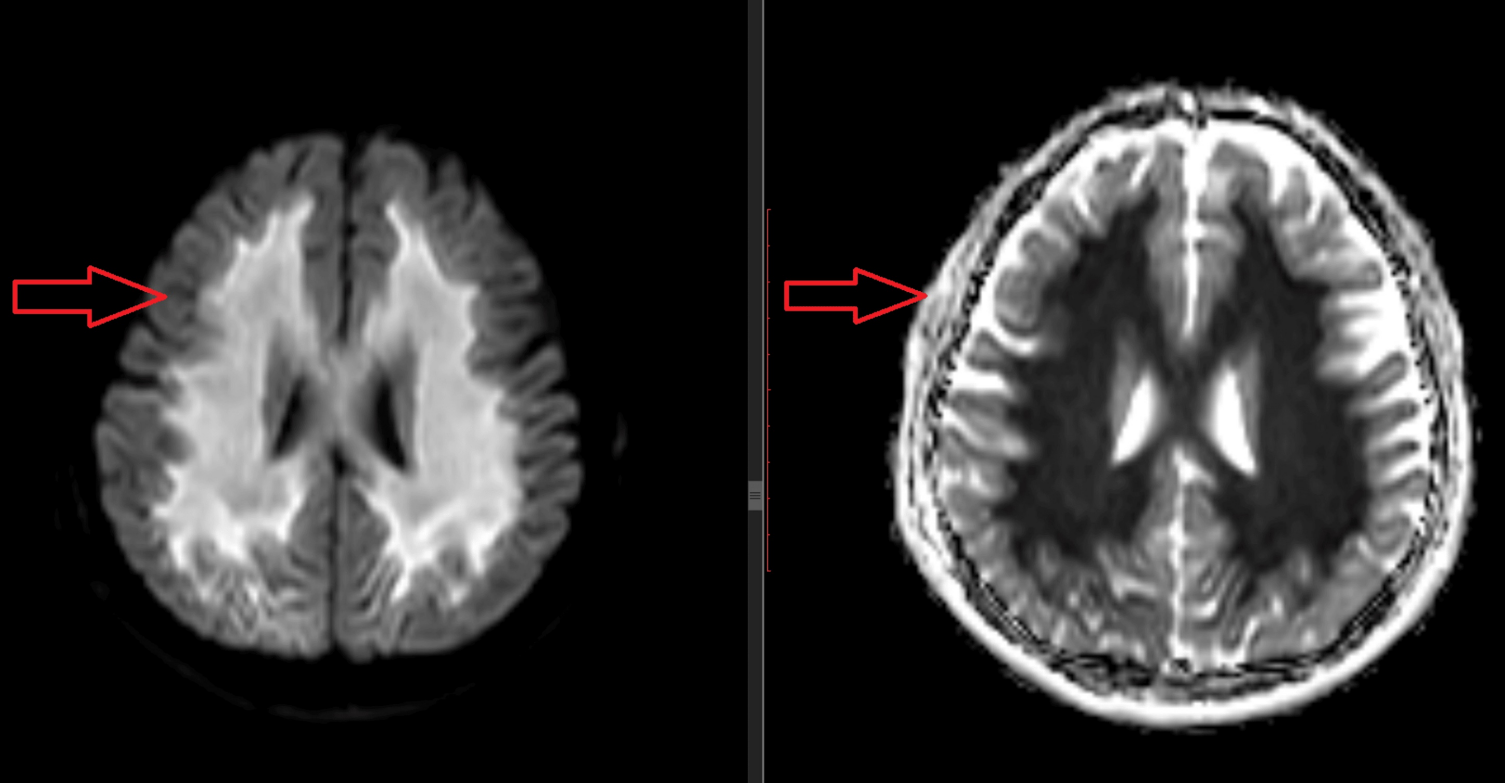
MRI diffusion-weighted imaging (DWI) (a) and apparent diffusion coefficient (ADC) (b) images (March 2024) shows progressive disease with increase in bilateral symmetrical periventricular white matter areas of diffusion restriction.
